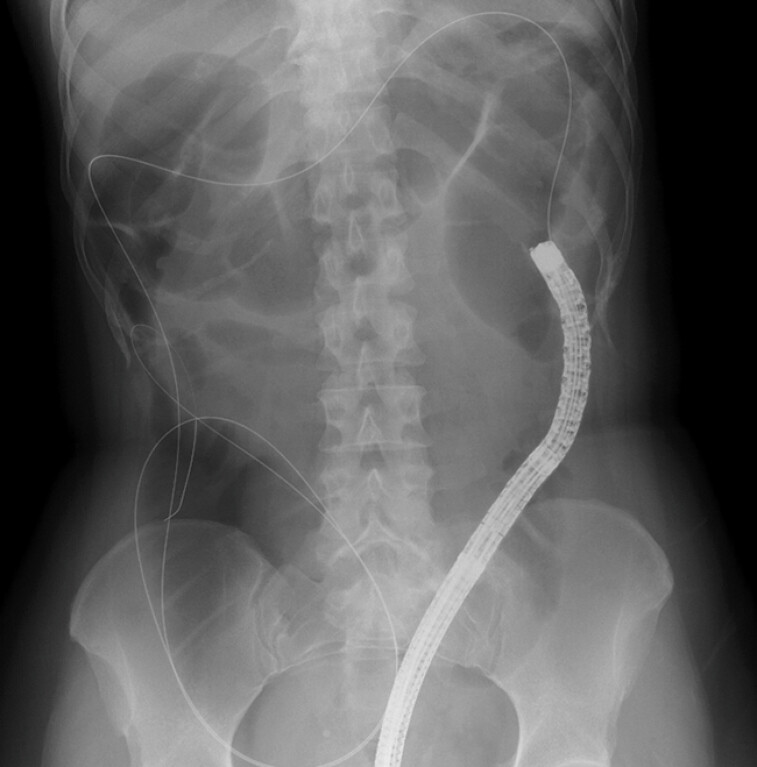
Radiographic image showing the inserted guidewire at the tip of the scope, which was confirmed, on imaging, as remaining in the cecum as the scope was carefully withdrawn.
